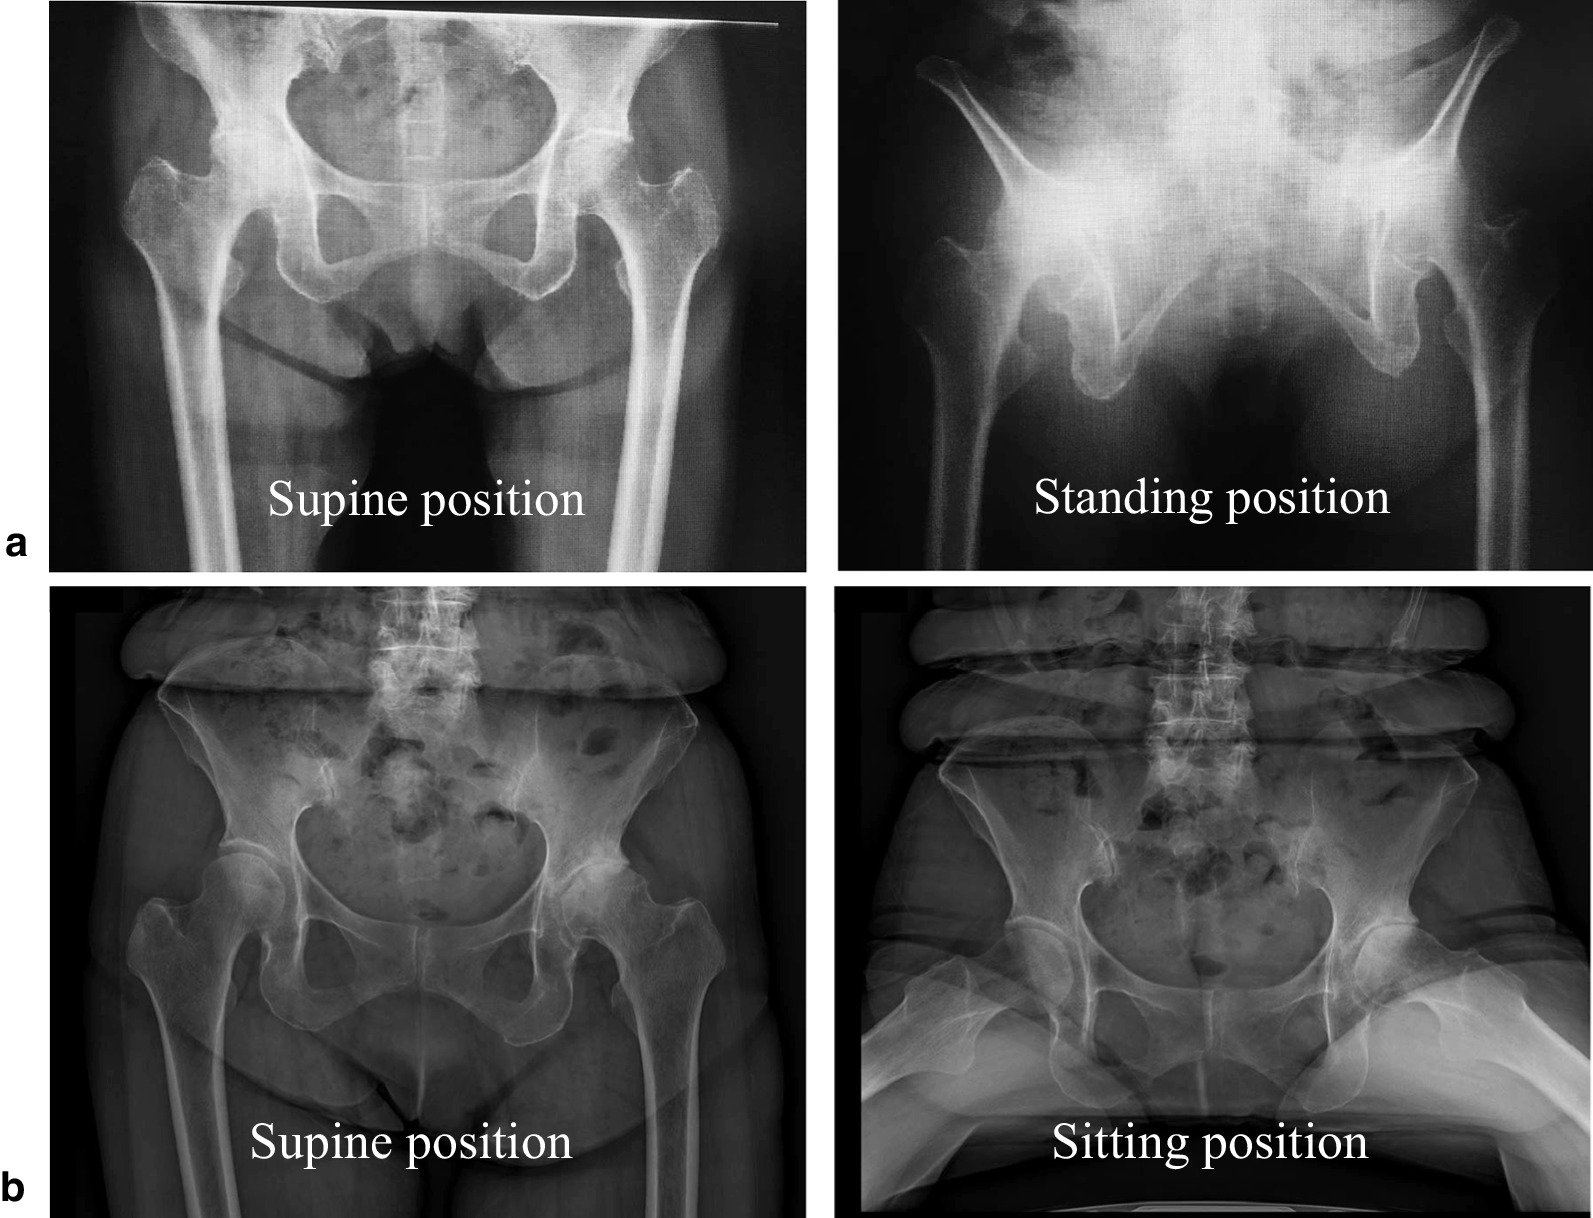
Pelvic postural change in the supine, standing, and sitting positions. a When the posterior tilt during supine to standing position is large, the target radiographic anteversion (RA) is 5° or 10°. b When the posterior tilt during supine to the sitting position is small, the target RA is 20° or 25°
